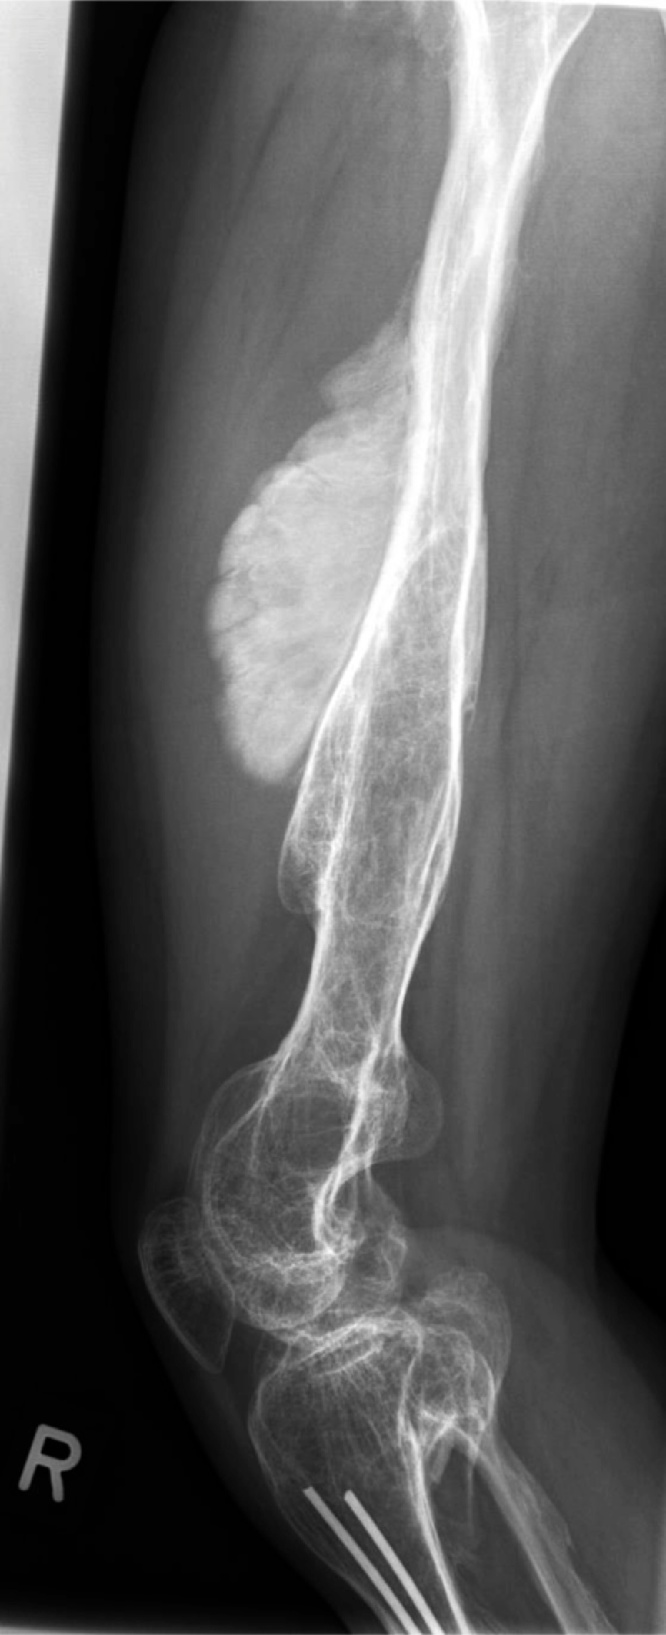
Radiographic imaging of the femoral shaft in anterior-posterior and lateral views showing a massive irregular periosteal hypertrophic lamellar ossification at with an irregular sunburst pattern as well as the appearance of spiculae. Furthermore the radiographs show the condition of intramedullary nailing for a tibial shaft fracture years ago.
